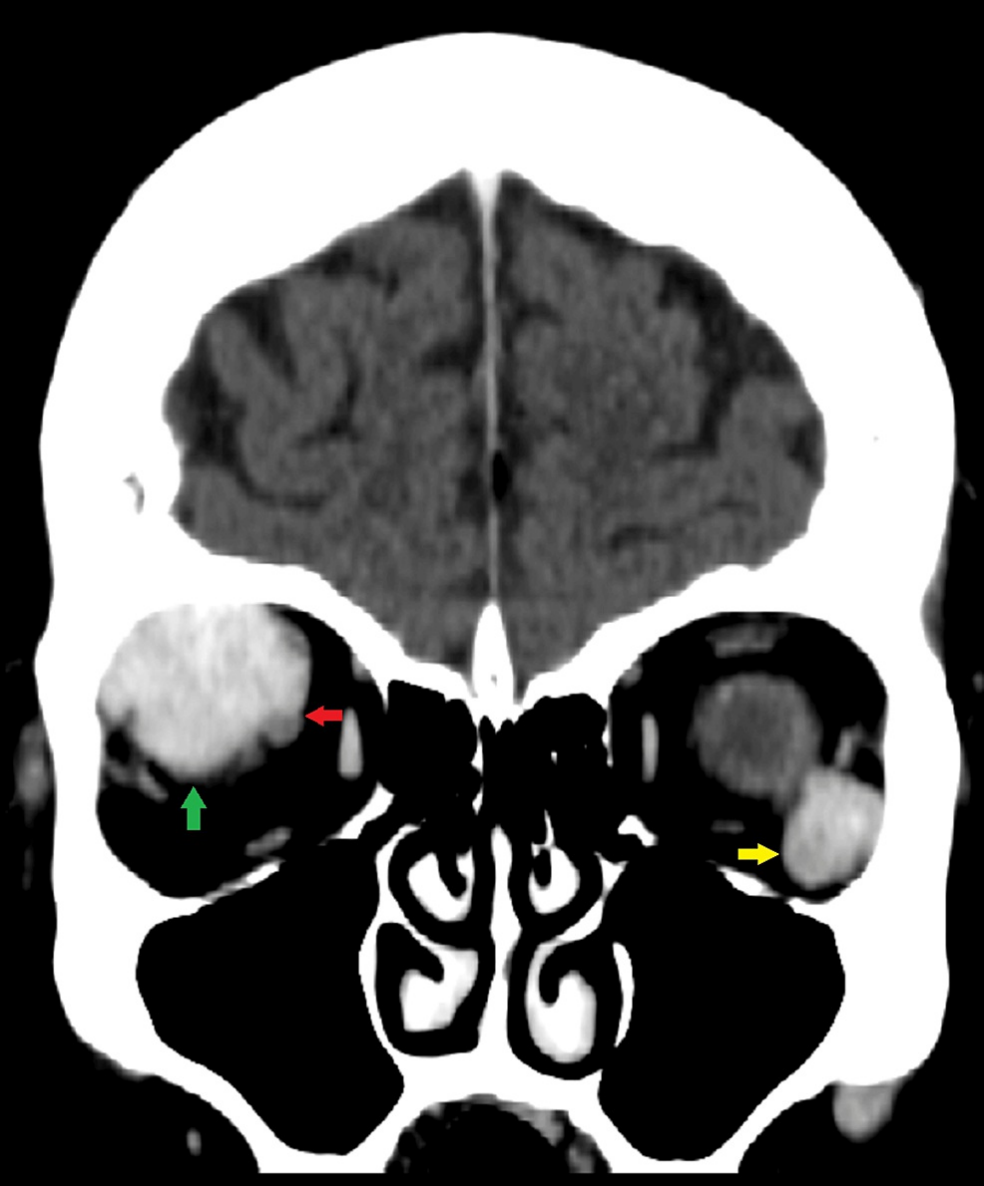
CT brain scan with contrast (coronal view) Well-defined, bilateral intra-orbital tumors with homogenous contrast enhancement are seen (green and yellow arrows). The lesion in the right orbit is encasing and compressing the right optic nerve (red arrow).
